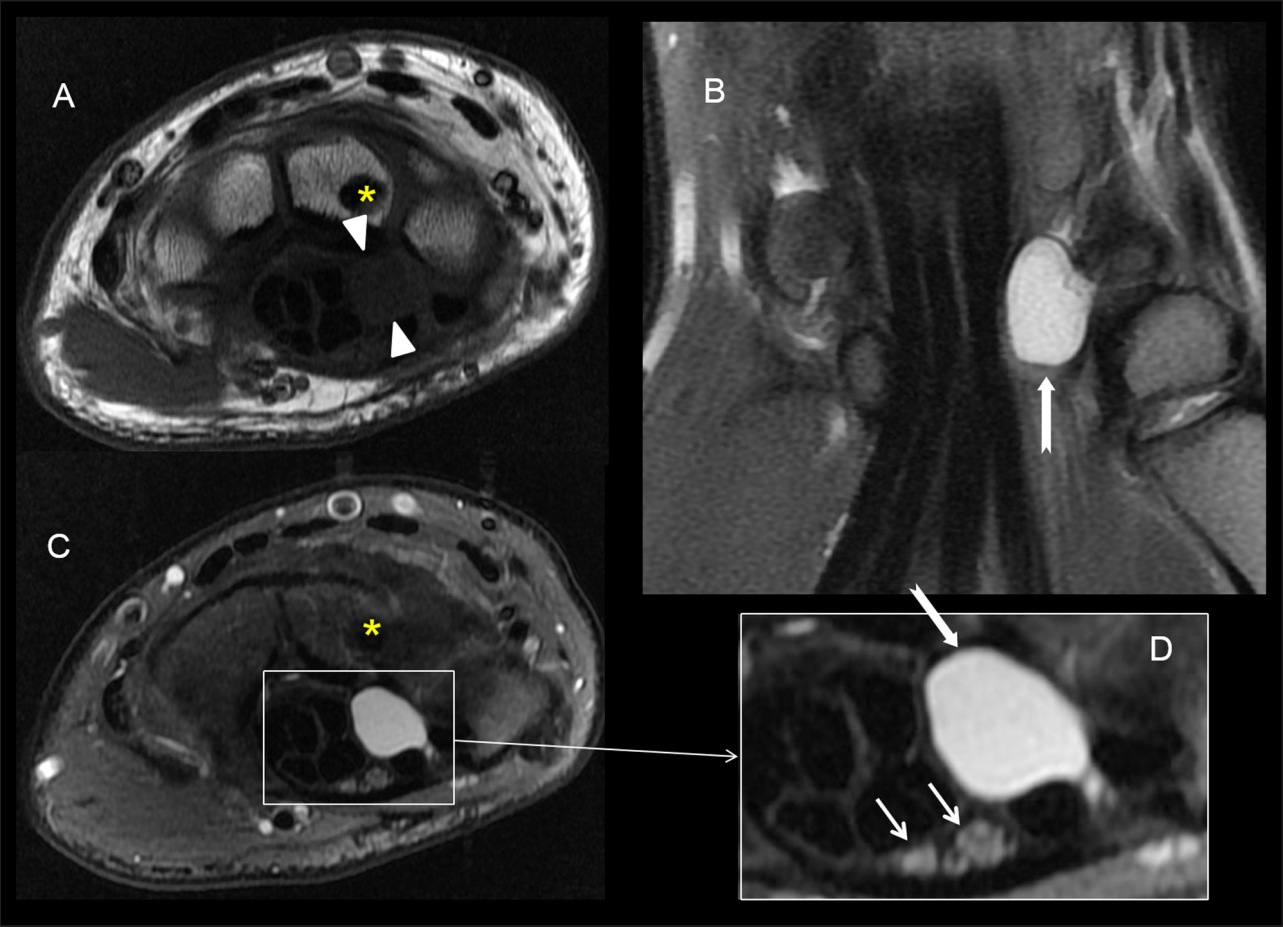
Median nerve compression due to a ganglion cyst originating from the joint. T1-weighted axial image A) and T2-weighted axial (C, D), coronal B) and sagittal E) images with Fat Sat. T1-weighted sagittal image with FS after injection of gadolinium (F). MRI showed a space-occupying mass with fluid-like signal (low signal on T1 (white arrowheads) and high signal on T2-weighted images (white arrows) located at the deep and lateral part of the carpal tunnel. It corresponded to a ganglion cyst originating from the ventral scapholunate interval. The cyst caused an extrinsic compression of the bifid median nerve (small white arrows) which showed an increased signal on T2-weighted images (yellow arrowheads) and an enhancement after the IV administration of gadolinium (yellow arrows). The ganglion cyst presented a discrete and peripheral enhancement (black arrowheads). Note the presence of an incidental enostosis (yellow asterisk).
